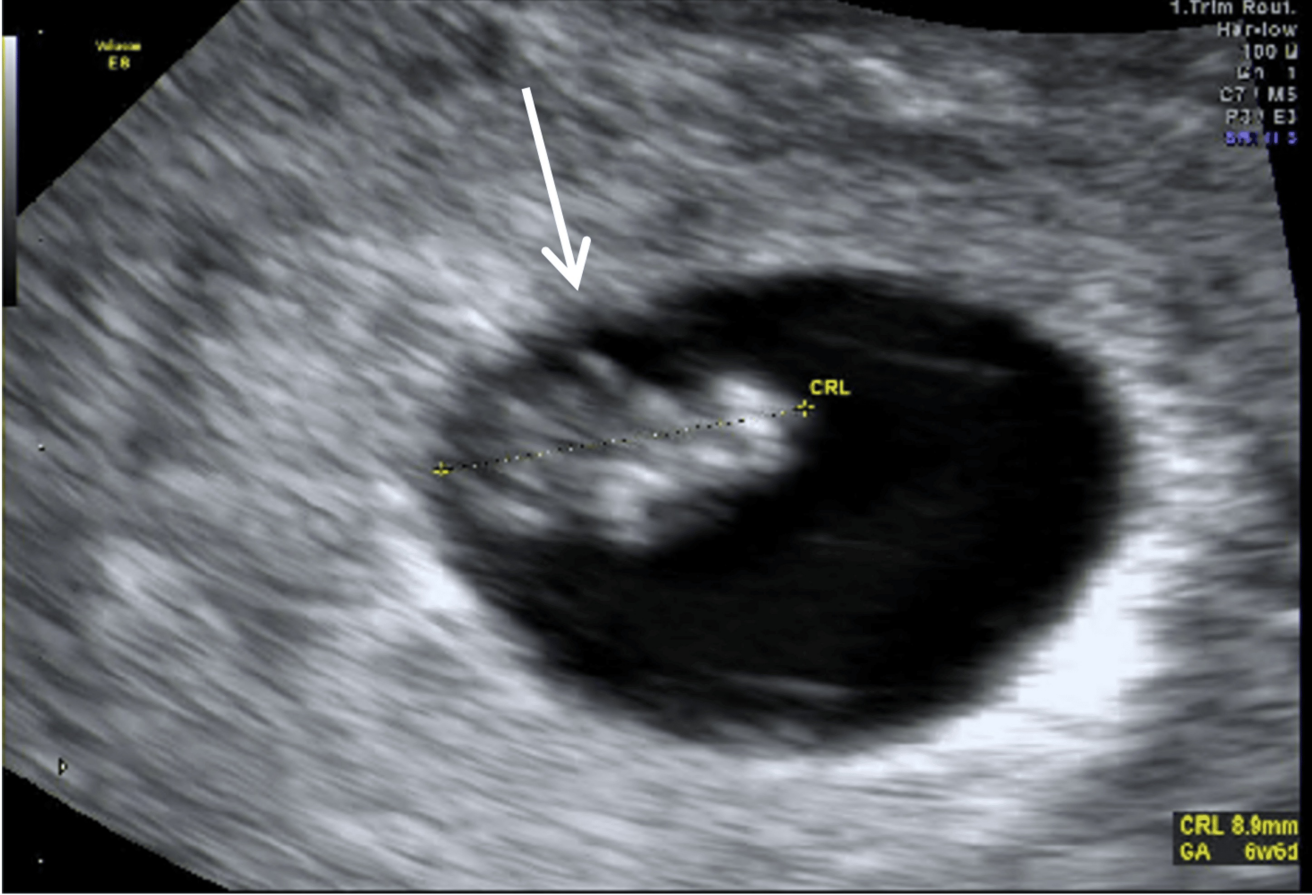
Follow-up transvaginal ultrasound 27 days after OPU The white arrow indicates an intrauterine gestational sac with a CRL corresponding to six weeks and six days. CRL, crown-rump length; GA, gestational age; OPU, oocyte pick-up
